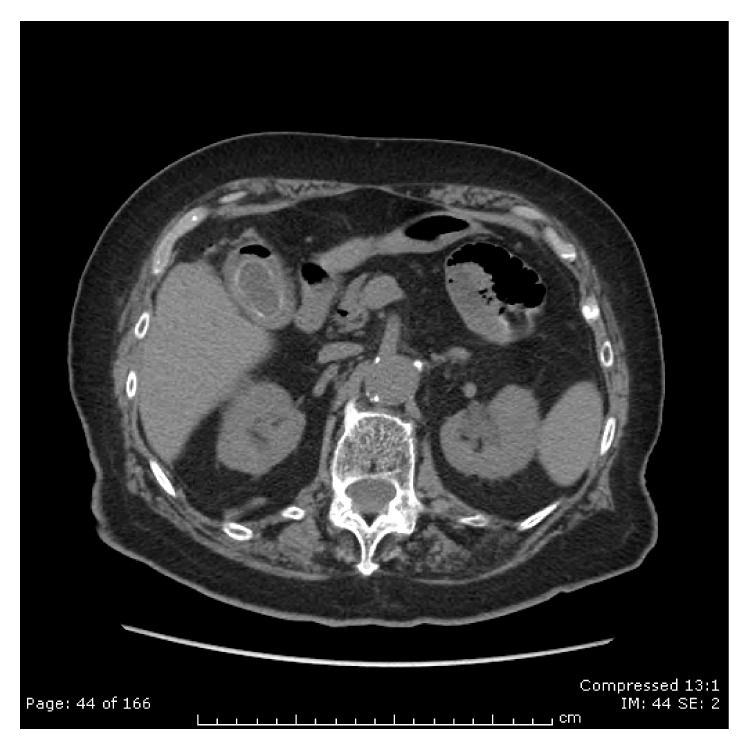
Computed tomography. Pneumobilia.
